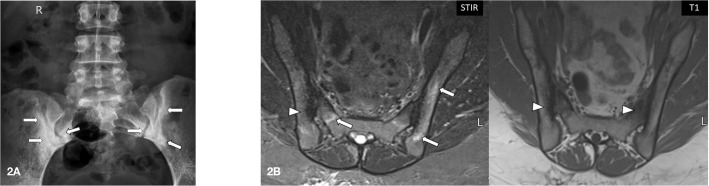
(A) Radiograph of the SIJ from a 25 yo female patient multipara with chronic lower backpain caused by OCI. Moderate paraarticular multisclerosis in the SIGs emphasized on both iliac sides without definite evidence of erosions or ankyloses. (B) MRI of the same patient. Oblique transversal STIR and T1-weighted images of the SIJ. Paraarticular bone marrow oedema, shown as hyperintense signal on STIR (white arrows) and paraarticular multisclerosis of the SIJ bilateral, sacrum and ilium side, without erosive changes, shown as hypointense signal on T1-weighted MR (white arrowheads).
